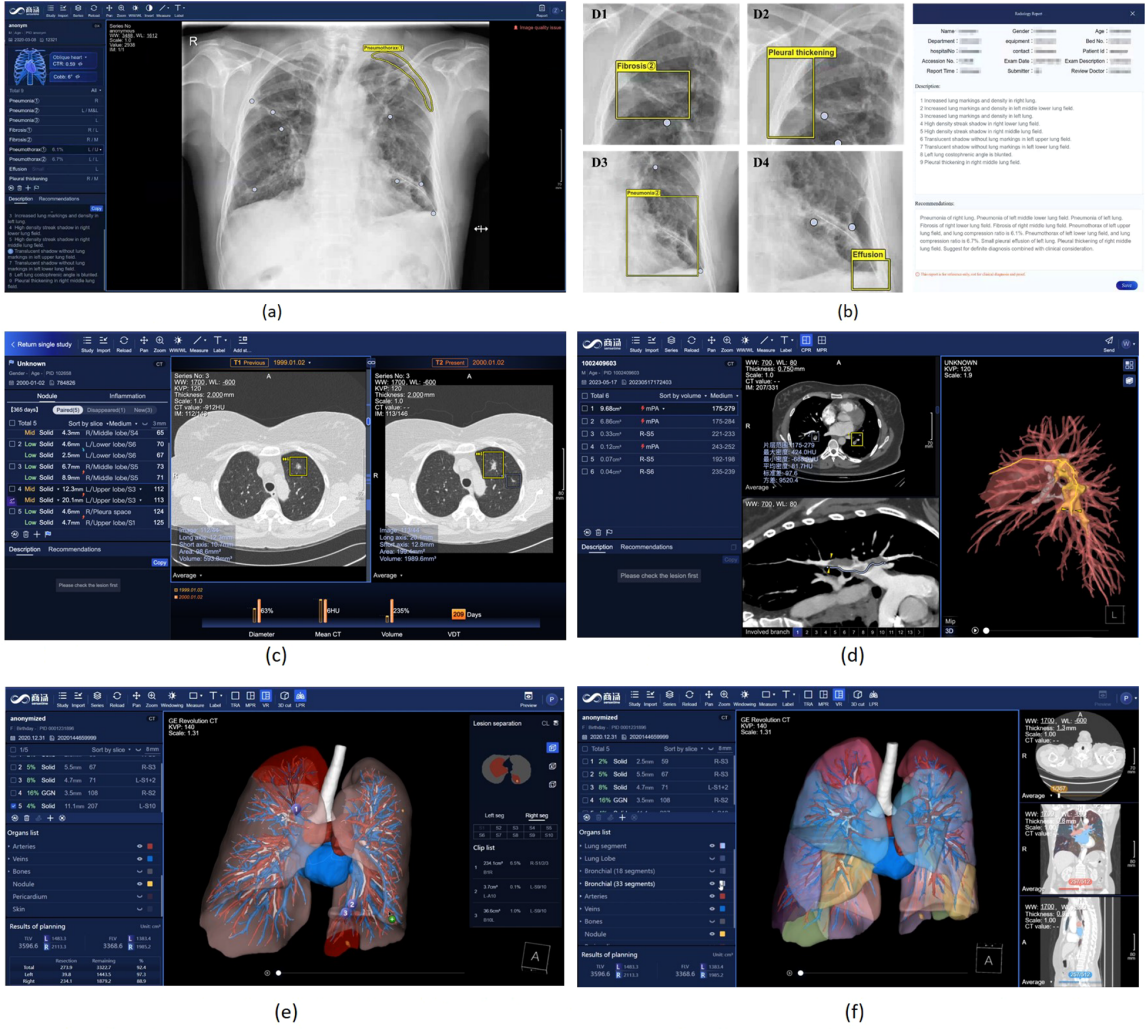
Chest X-Ray image and Lung CT analysis in SenseCare. (a) User interface of Chest X-ray analysis. (b) Multi-disease detection and automated report generation. (c) Intelligent lung nodule analysis and follow-up in CT. (d) Intelligent analysis of pulmonary embolism. (e) Lung surgical planning with visualized traced tube structures. (f) Lung surgical planning with lung segment analysis.
